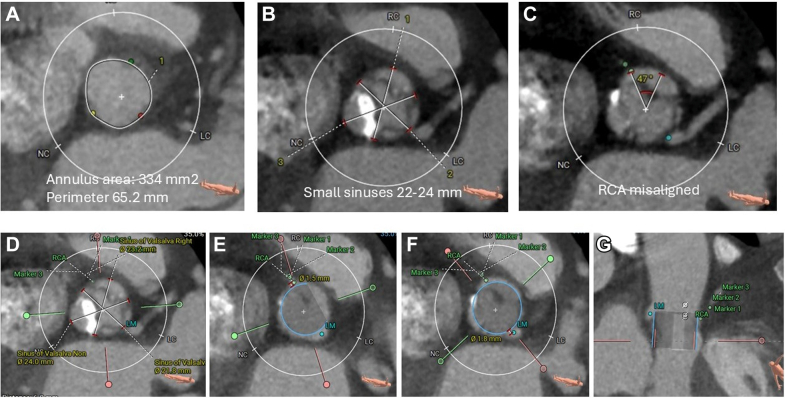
Case 2: Preprocedural Computed Tomography Angiography and Virtual Modeling of the Transcatheter Heart Valve (A to D) Preprocedural computed tomography angiography showing high-risk features: small annulus (A) and sinuses of Valsalva (B), with the RCA arising off axis in the right coronary cusp (C and D). (E to G) VTC distance of <2 mm was found for both the LM and RCA when a 20-mm Sapien S3 virtual valve was modeled. Abbreviations as in Figure 1.
